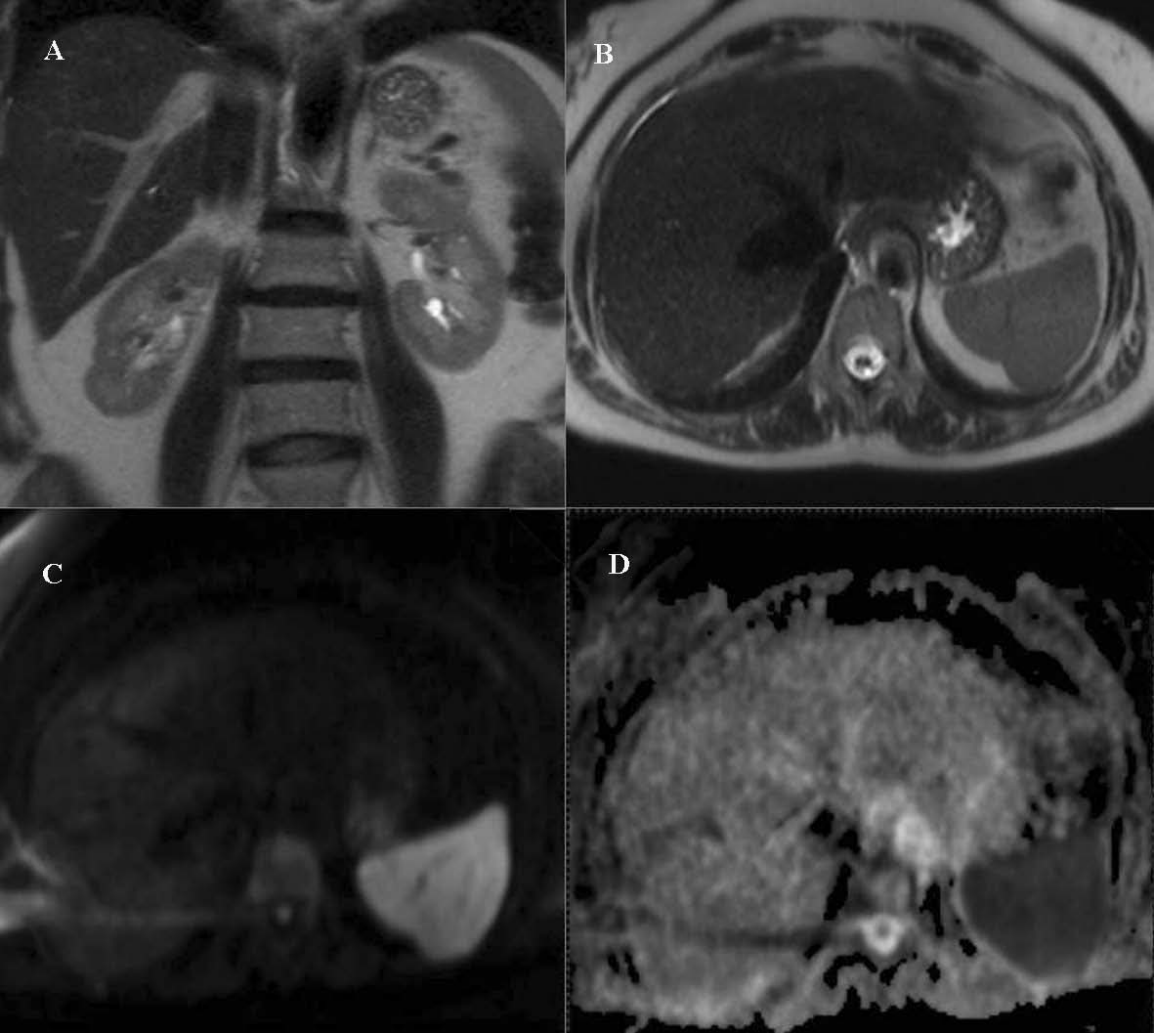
MRI sequences of a patient with moderate PHT (43 year old female). There is slight enlargement of IVC and hepatic veins. A Coronal T2-weighted sequence, B Axial T2-weighted sequence C Axial diffusion-weighted image (b = 1000 s/mm^2^), D ADC map of the same patient; calculated ADC was 1.86 × 10ˉ³ mm²/s (increased diffusion due to intercellular fluid accumulation that is not apparent visually, however detectable with ADC calculation).
